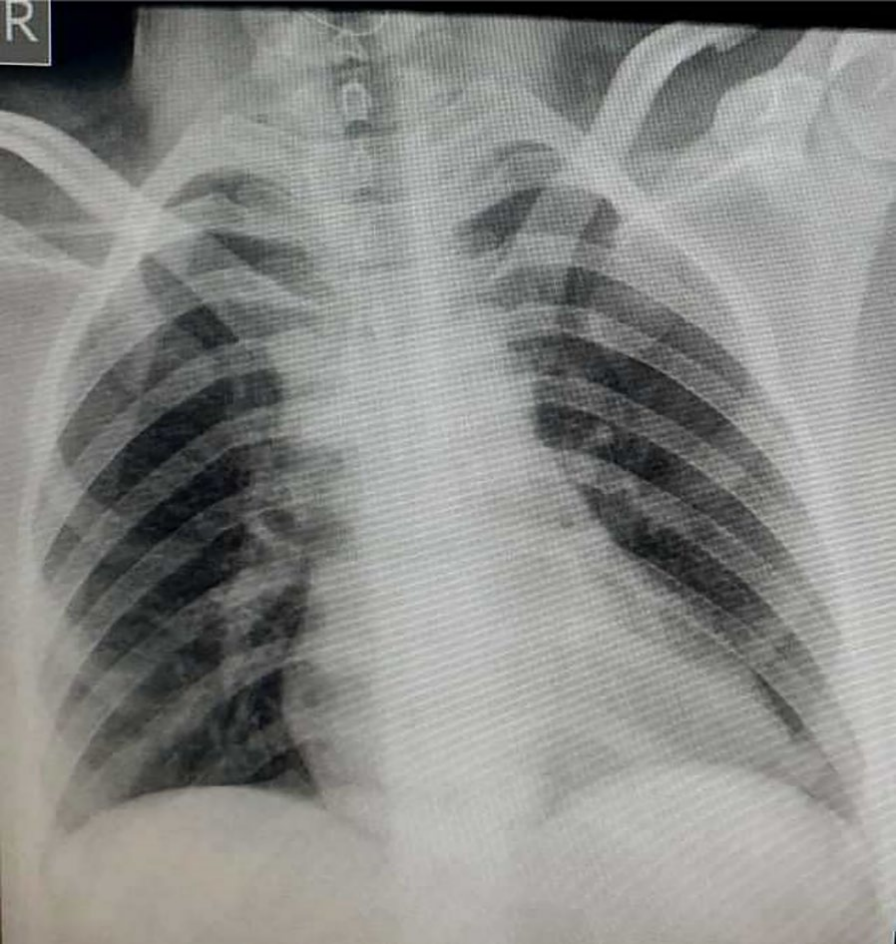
Posteroanterior chest X-ray view with normal lung appearance
